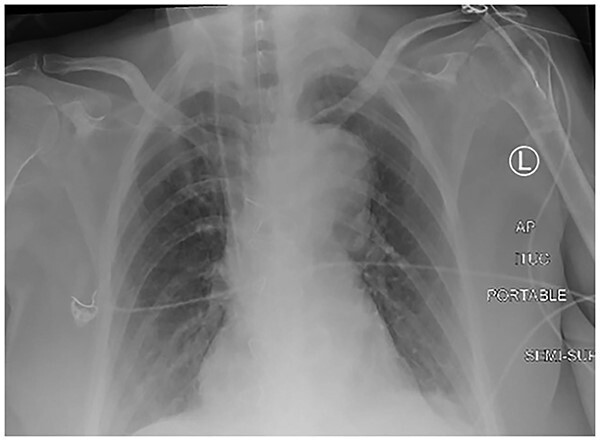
Erect chest X-ray demonstrating left pleural effusion and an unfolded, dilated aortic arch.
